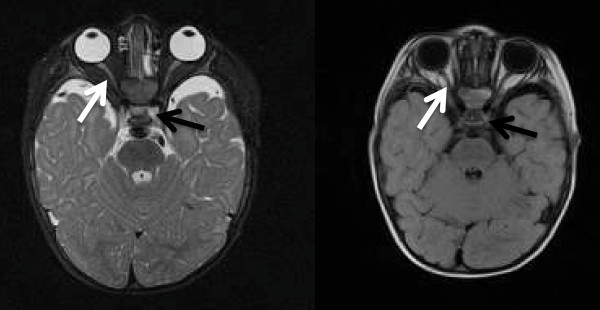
Axial Tx and Ty MRI scans showing complete absence of the optic chiasm (black arrow); the optic nerves can be identified only in the intraorbital portion (white arrow).
